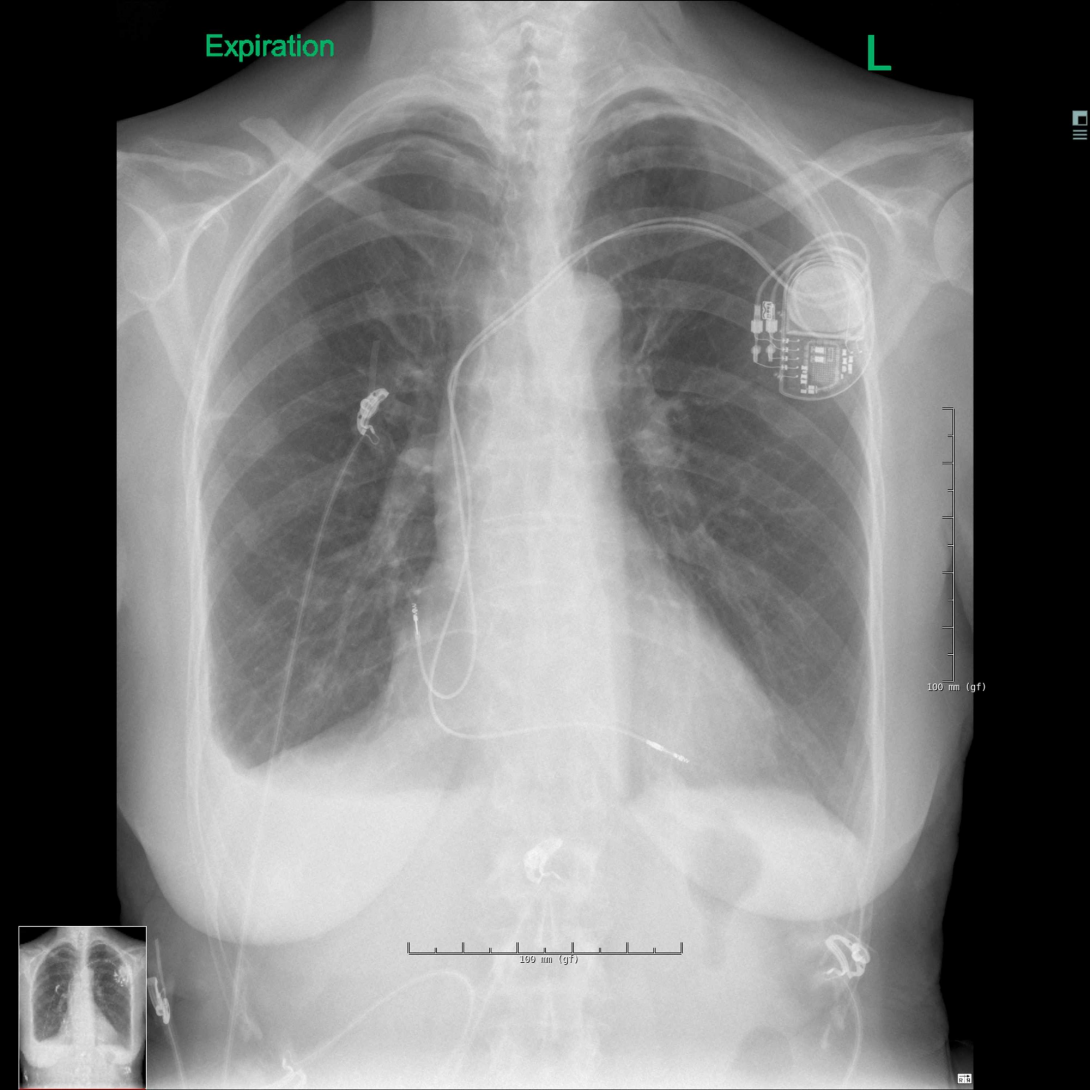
Chest X-ray during expiration on day 3.
